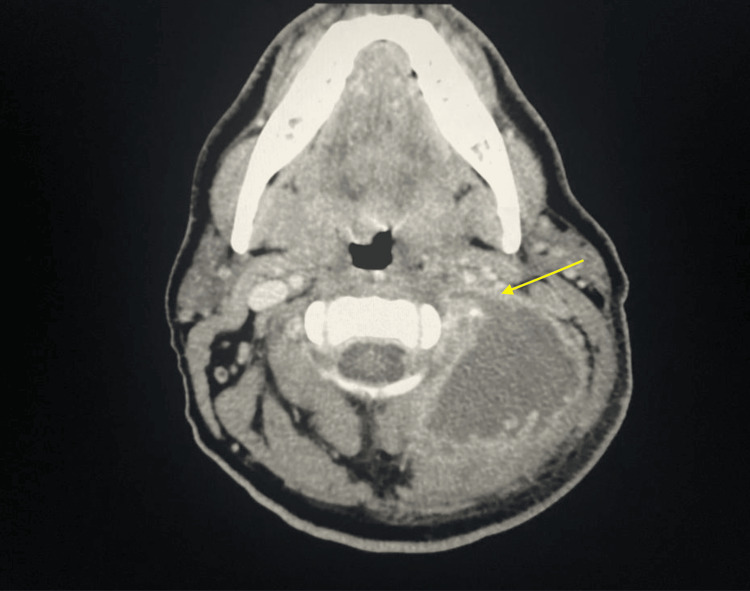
Non-contrast axial neck soft tissue CT scan demonstrating occlusion of neck vessels
